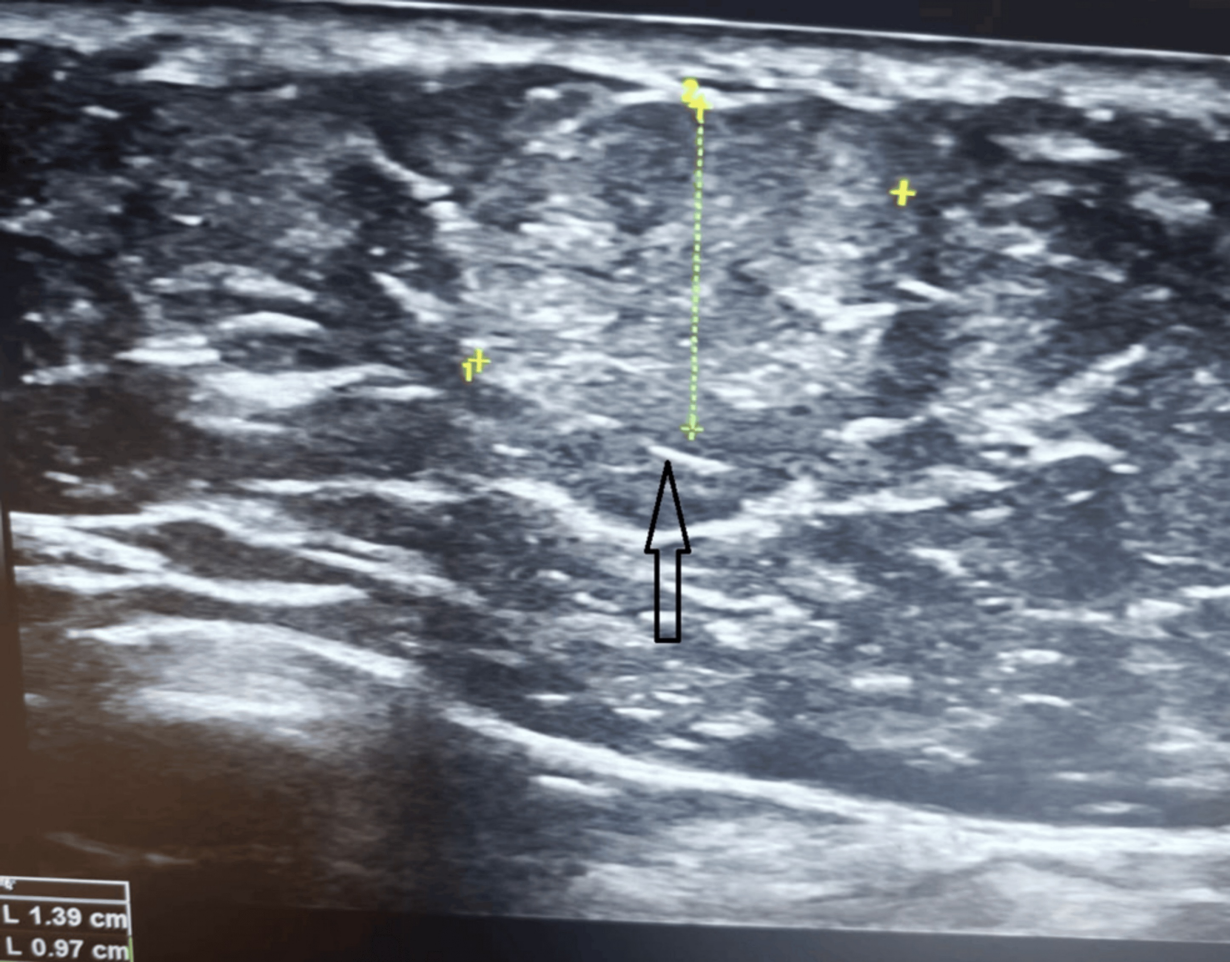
Targeted ultrasound of the right hypochondrium showing a well-defined subcutaneous lipoma (arrow) within the abdominal wall at the site of maximal tenderness.
